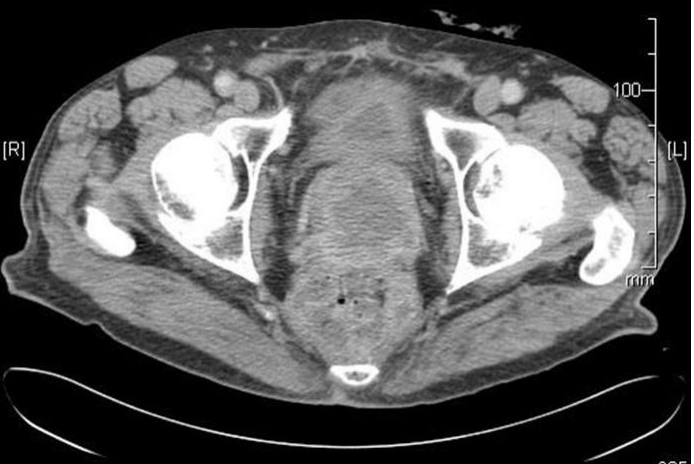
CT Scan of the Pelvis showing prostatic mass.
